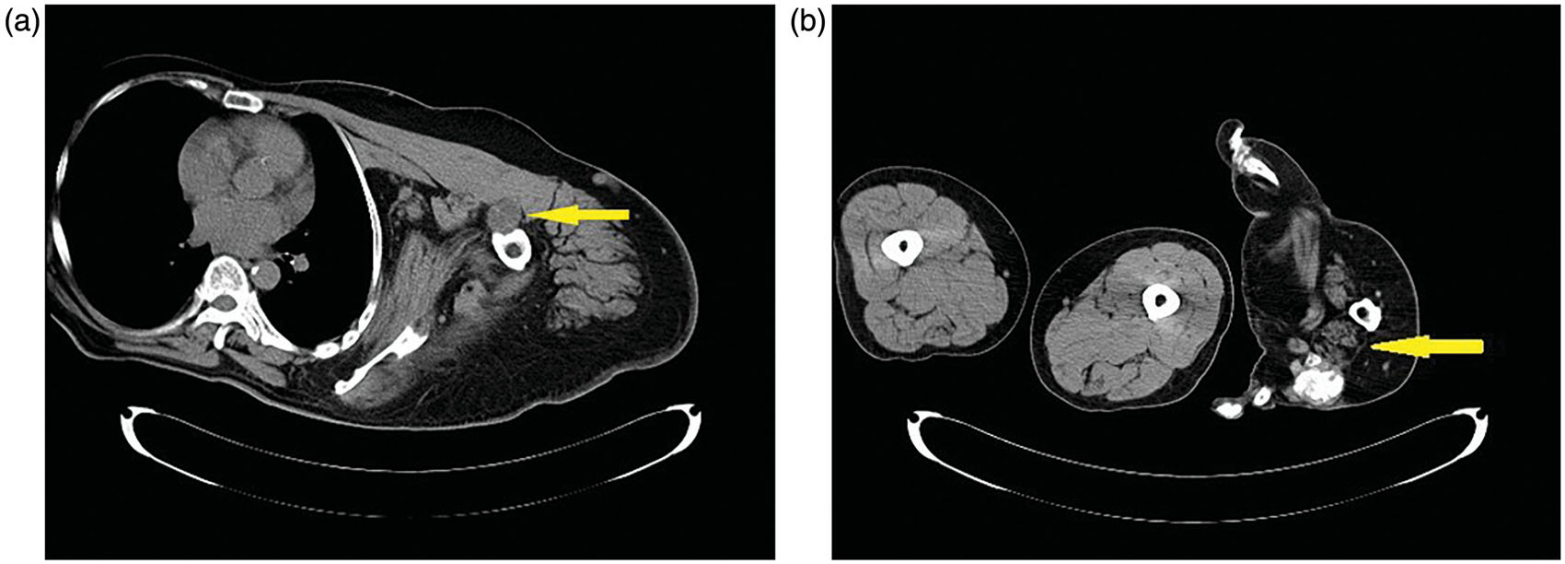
(a,b) MRI: MRI showed increased T2 signal in the interior of the median nerve, suggesting the presence of fat. The median nerve was enlarged of both upper extremities. Especially, adipose hyperplasia was salient in the carpal tunnel.
